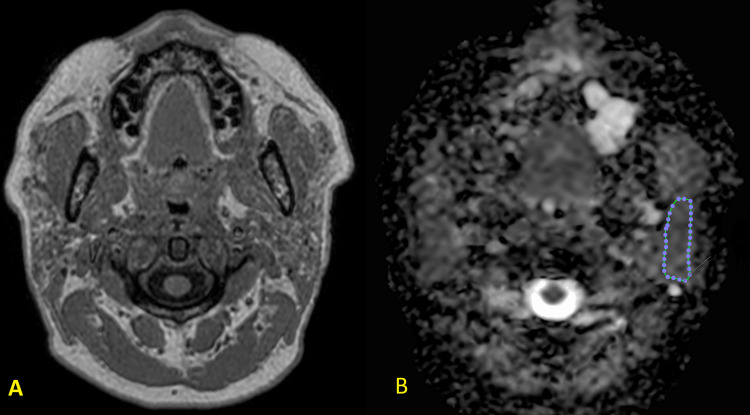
ADC measurement on the left parotid gland. (A, left) T1-weighted image depicts heterogeneity due to grid-like fat deposition in the parotid glands (Rao grade 2). (B, right) A region of interest manually drawn over the left parotid gland returned a mean ADC of 0.815 × 10⁻³ mm²/s. ADC: apparent diffusion coefficient
